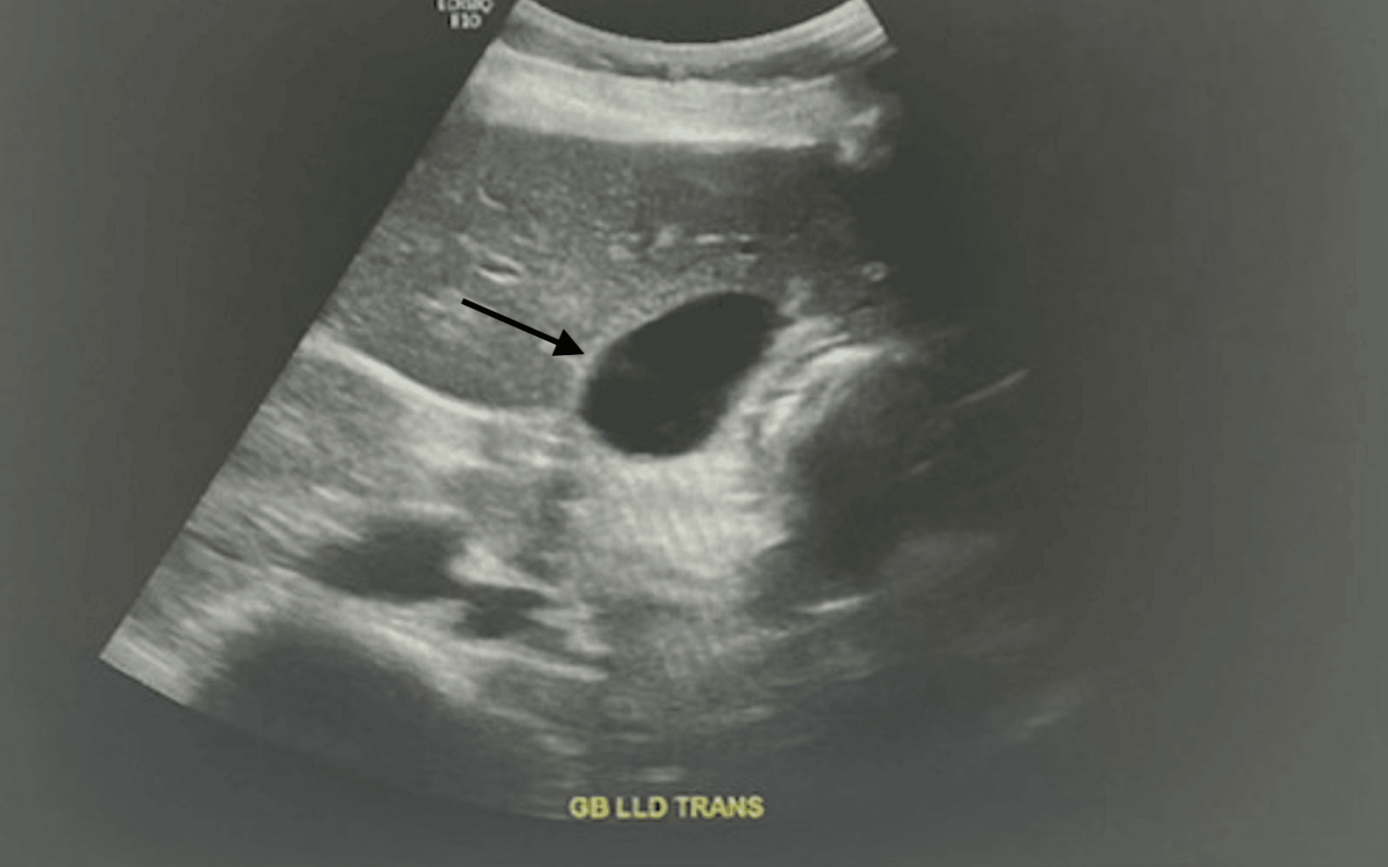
US abdominal Trace gallbladder sludge with borderline thickened gallbladder wall, no pericholecystic fluid, and negative sonographic Murphy sign; these findings are equivocal for acute cholecystitis. The follow-up HIDA scan was negative. US: ultrasound, HIDA: hepatobiliary iminodiacetic acid
